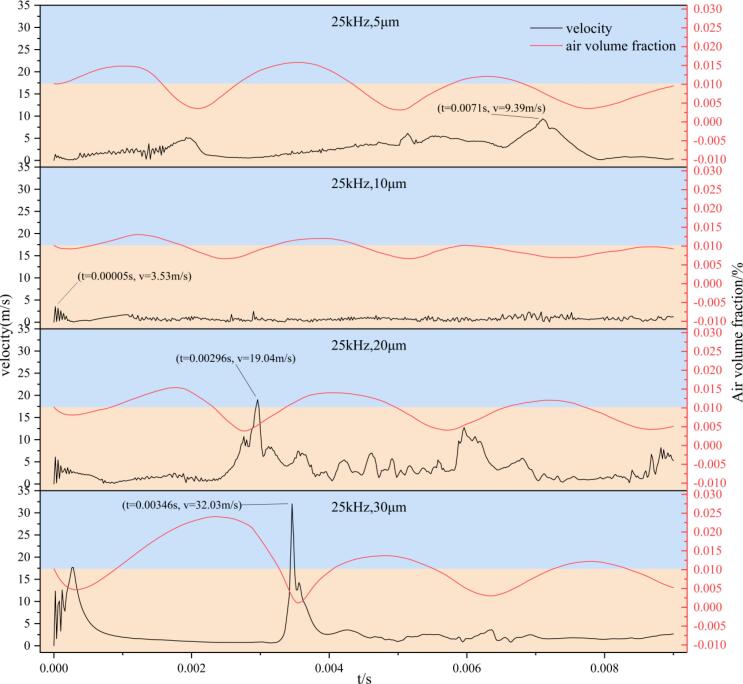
Temporal evolution of fluid velocity and air volume fraction at the monitoring point under different vibration amplitudes at an ultrasonic frequency of 25 kHz. The black curves represent the local fluid velocity (left axis), while the red curves denote the air volume fraction (right axis). From top to bottom, the panels correspond to vibration amplitudes of 5, 10, 20, and 30 μm, respectively. The numerical labels indicate the peak velocity values and their corresponding time instants.
